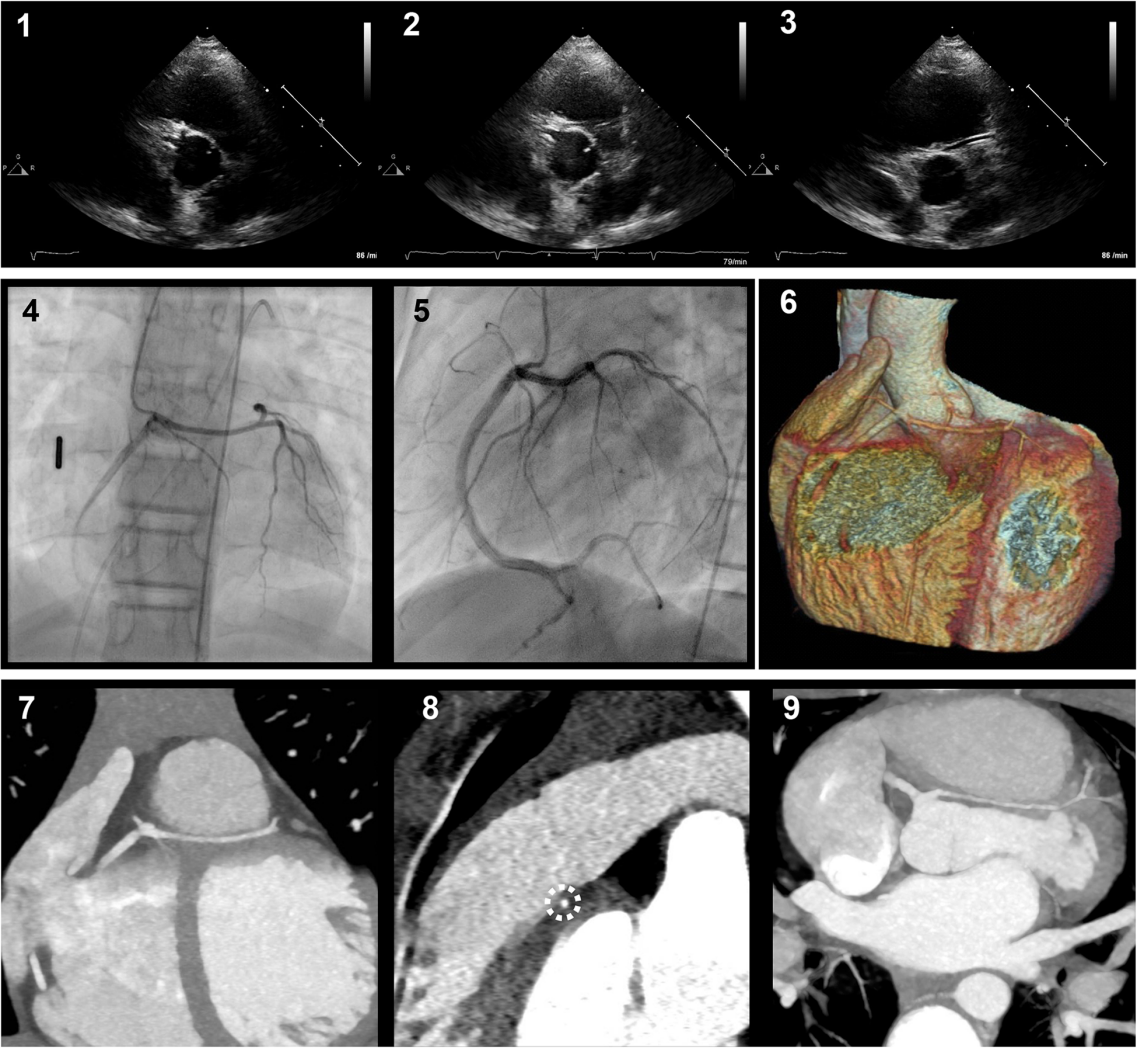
1-3 Echocardiography in the parasternal short axis view, showing origin of both coronary arteries from the right aortic sinus; 4-5 Selective coronary angiography confirming AAOLCA from the right aortic sinus; 6 Cardiac CT-scan with prospective gating, in coronal plane showing AAOLCA from the right aortic sinus taking a subpulmonic intramyocardial course, volume rendering technique (VRT), AW-Server 2.0; GE, General Electric Company, Boston (USA); 7-9 Cardiac CT-scan in the coronal, sagittal and transversal plane showing AAOLCA from the right aortic sinus taking a subpulmonic intramyocardial course (circle) below the right ventricular outflow tract (syngo.via; Siemens Healthcare GmbH; Erlangen, Germany)
